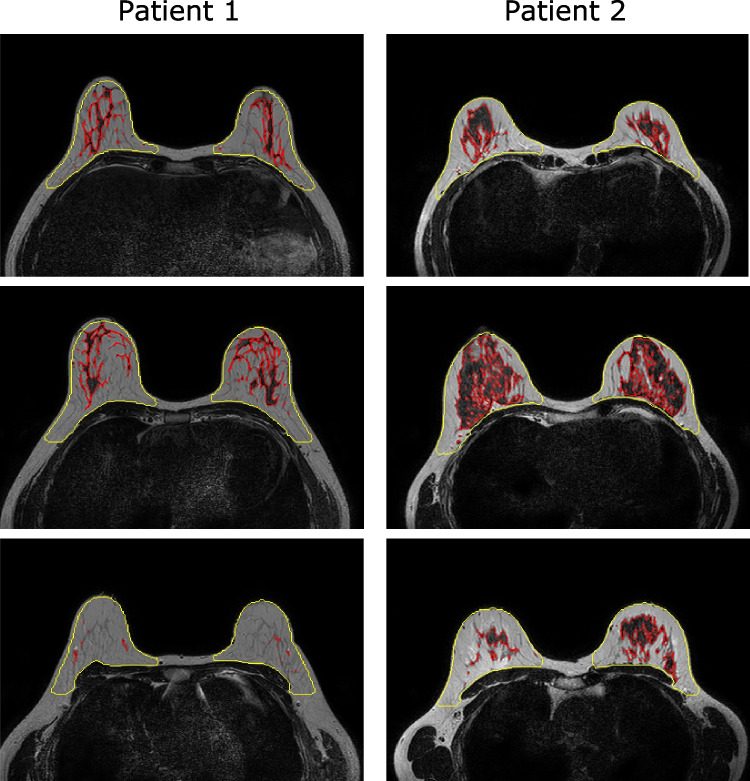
Semi-automatically generated breast masks (yellow) and parenchyma segmentations (red) overlayed on three representative axial slices of T2-weighted breast scans from two example patients. Patients were scanned at site 1 (left column) and site 2 (right column). Inferior slice locations are on the top row, while superior locations are on the bottom row. Images are displayed according to radiological convention (right breast is on left side of images)
